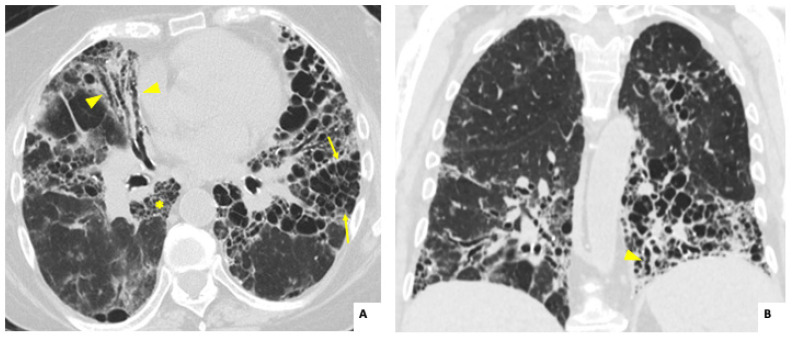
Axial (A) and coronal (B) high-resolution computed tomography images showing extensive fibrotic interstitial lung disease in a 70-year-old woman, diagnosed with isolated ANCA-ILD and anti-MPO positivity. Note the basilar predominance of honeycombing (arrows), traction bronchiectasis (arrowheads), and reticulation (asterisk); all findings are consistent with the usual interstitial pneumonia (UIP) pattern.
